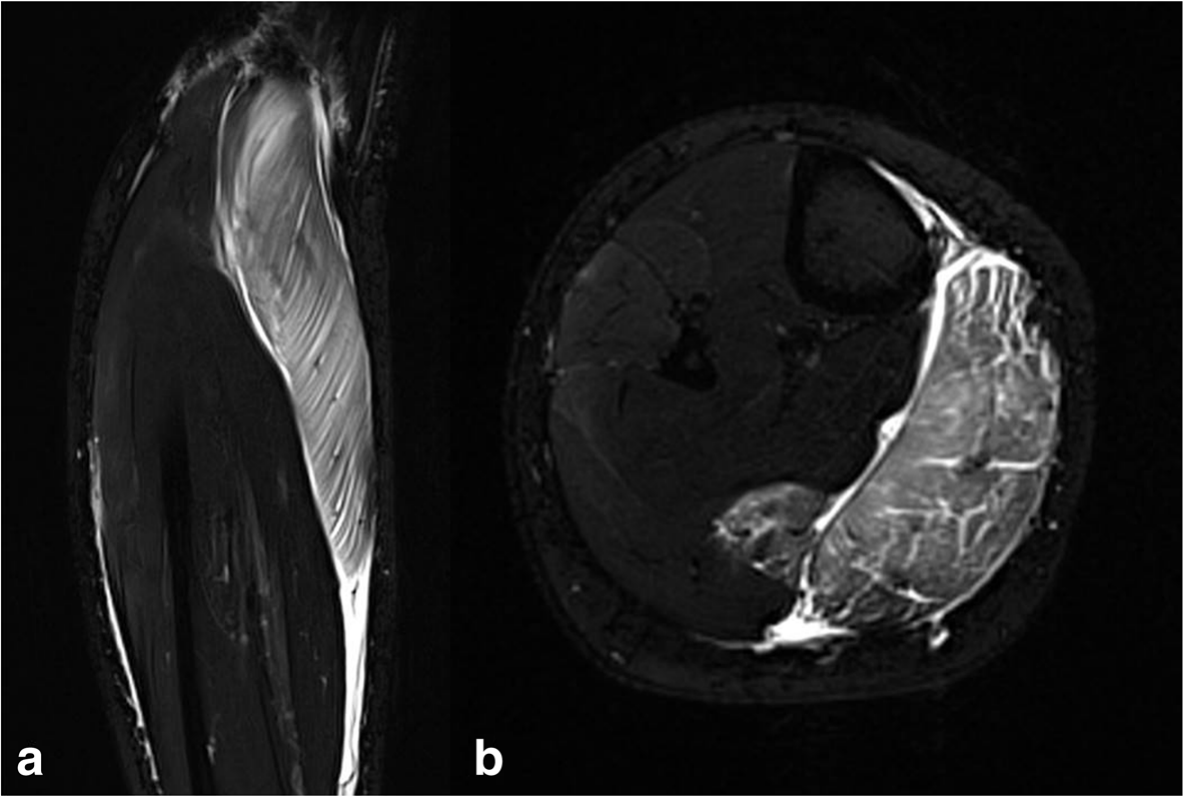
Indirect ultrastructural muscle injury (Type 1B: Delayed-onset muscle soreness (DOMS)). The MRI demonstrates a widespread intramuscular oedema corresponding to a raise in T2 signal intensity. a: Axial T2-weighted TIRM (Turbo-Inversion Recovery-Magnitude) sequence (total acquisition time, 3:31 min; inversion time, 260 ms; echo time, 69 ms; repetition time, 5120 ms; flip angle, 145°; resolution, 0.8 × 0.8 × 4.0 mm). b: coronal T2-weighted TIRM sequence (total acquisition time, 3:42 min; inversion time, 260 ms; echo time, 68 ms; repetition time, 5120 ms; flip angle, 180°; resolution, 0.9 × 0.9 × 4.0 mm). Published by kind permission of the Department of Radiology, University Hospital Erlangen
